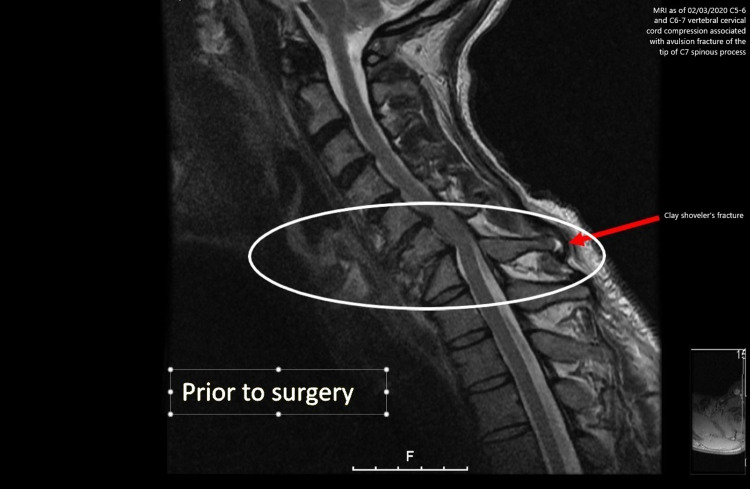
MRI scan indicates C5-6 and C6-7 vertebral cervical cord compression associated with an avulsion fracture of the tip of the spinous process. MRI: magnetic resonance imaging
